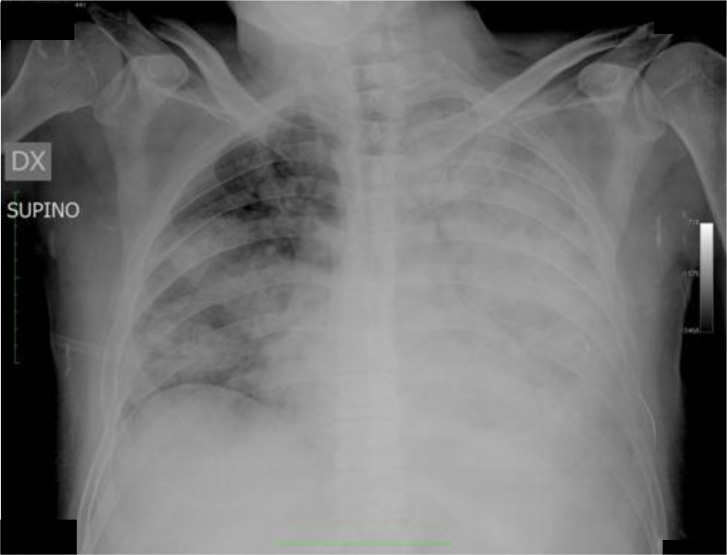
Chest x-ray at the admission in ER. Evidence of large bilateral opacities.
